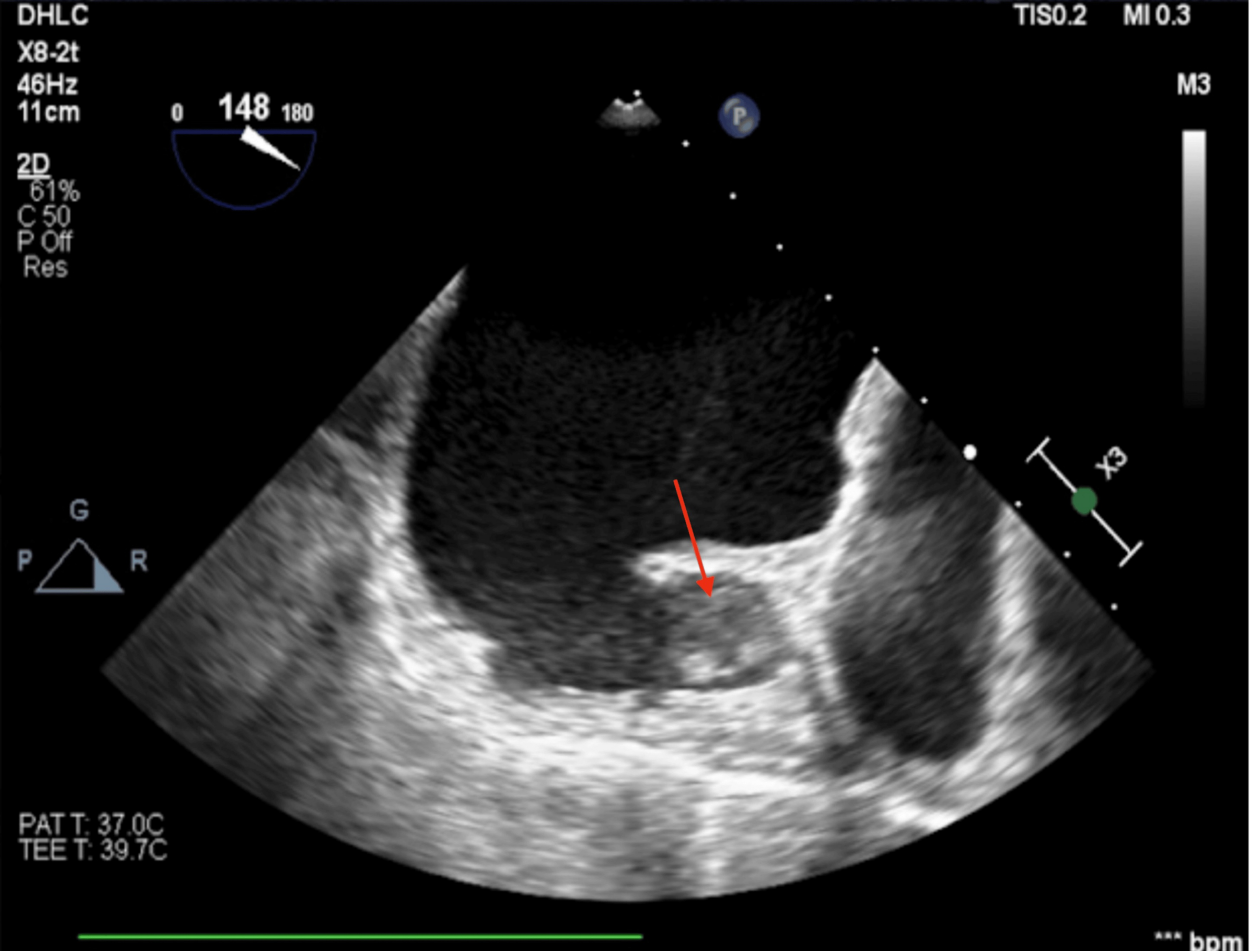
Two-dimensional transesophageal echocardiography (TEE) image of the left atrial appendage showing a 2.5 cm echodensity (red arrow) consistent with thrombus
